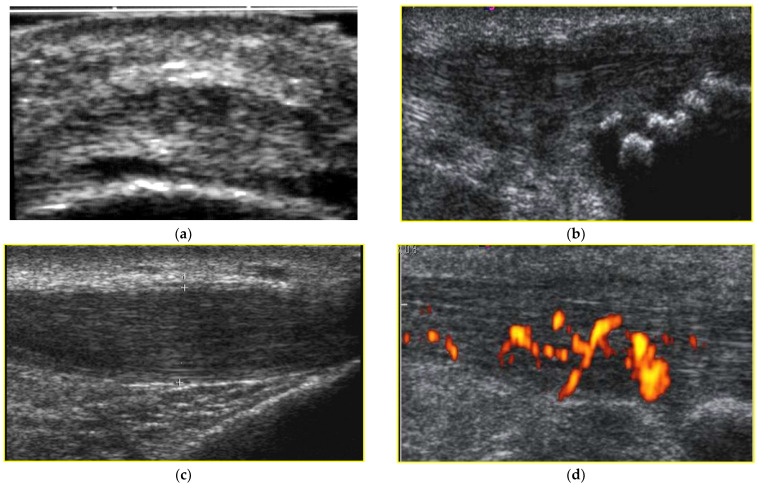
(a) Echostructural alterations (grade 2), (b) insertional tendon calcifications, (c) echostructural alterations (grade 1), and (d) signs of hyperemia on power color Doppler.
